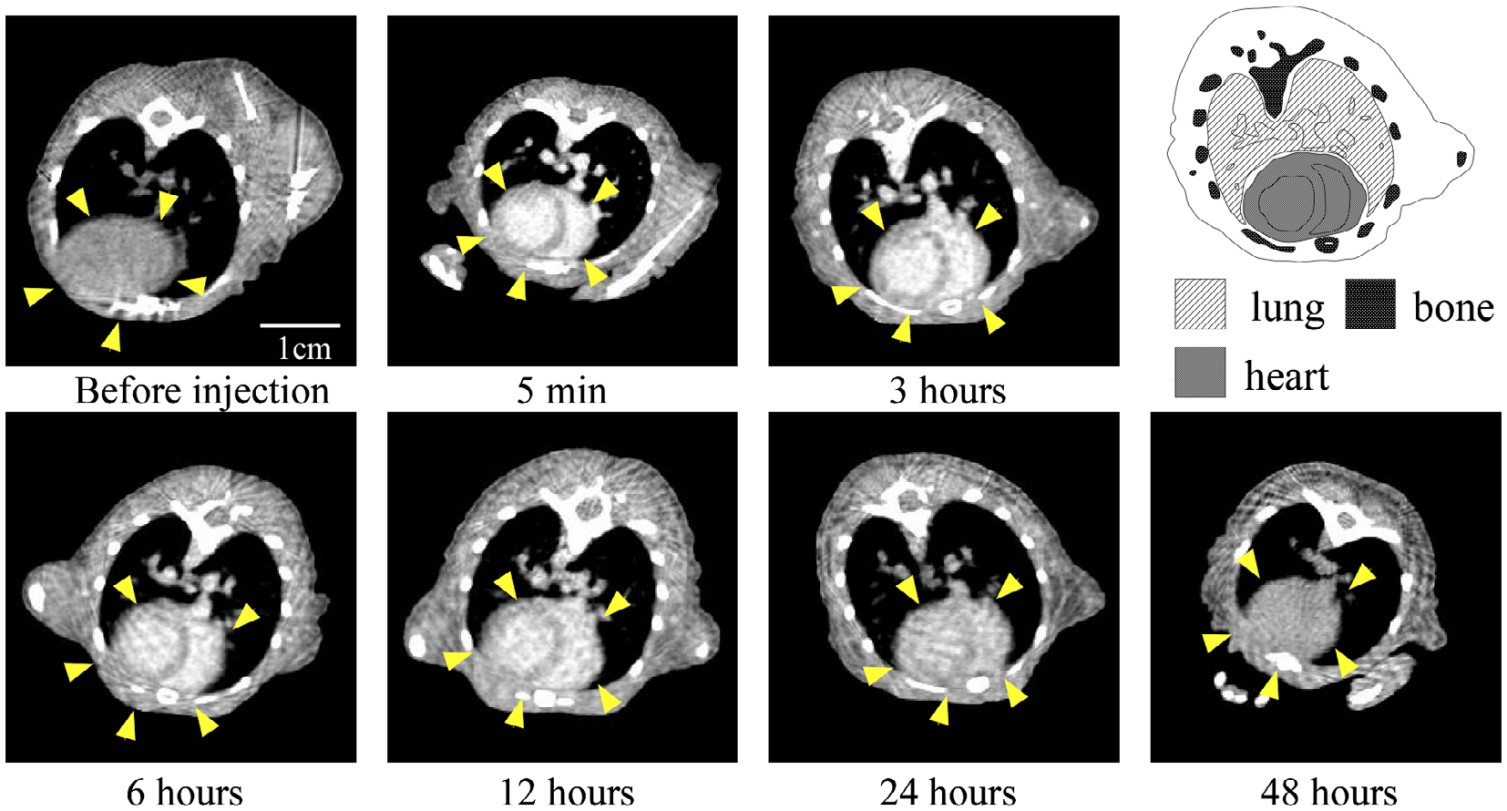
Change in CT value of a heart slice before and after injection of 30-nm Au-PEG. Yellow arrowheads indicate the region of the heart.
